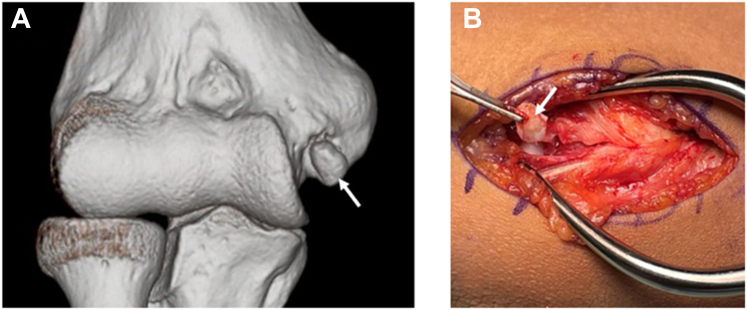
Pre-operative and intraoperative images. (A) Pre-operative 3D-CT image showing a chronic avulsion-type bone fragment at the proximal UCL attachment site (*white arrow*). (B) Intraoperative image after splitting the UCL along the direction of its fibers, showing the excised proximal bone fragment (*white arrow*). *3D*, three dimensional; *CT*, computed tomography; *UCL*, ulnar collateral ligament.
